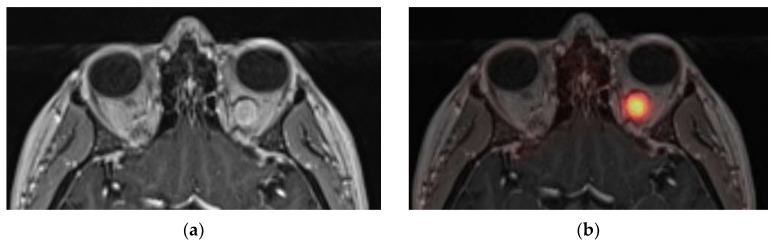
Case 4. (a) Axial MRI T1WI (a) showing outer enhancement of the left intraorbital tumour and axial fused MRI. (b) ^68^Ga-DOTATOC PET images showing intense tracer uptake in the left intraorbital tumour: tumour SUV_max_ = 19.6; pituitary SUV_max_ = 17.0.
